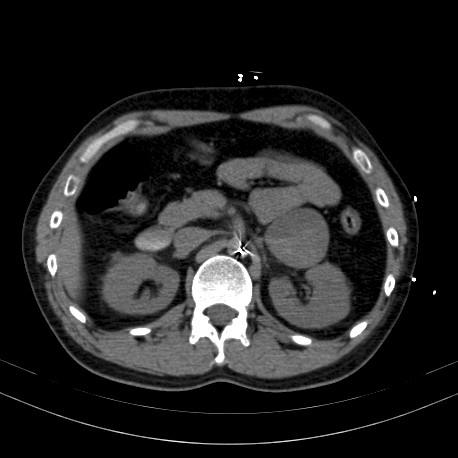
Abdominal CT scan: a solid mass measuring 5.5 × 5 × 4 cm is visible, touching the left adrenal gland and the cauda pancreatis; the mass contains varying focal densities consistent with bleeding.
